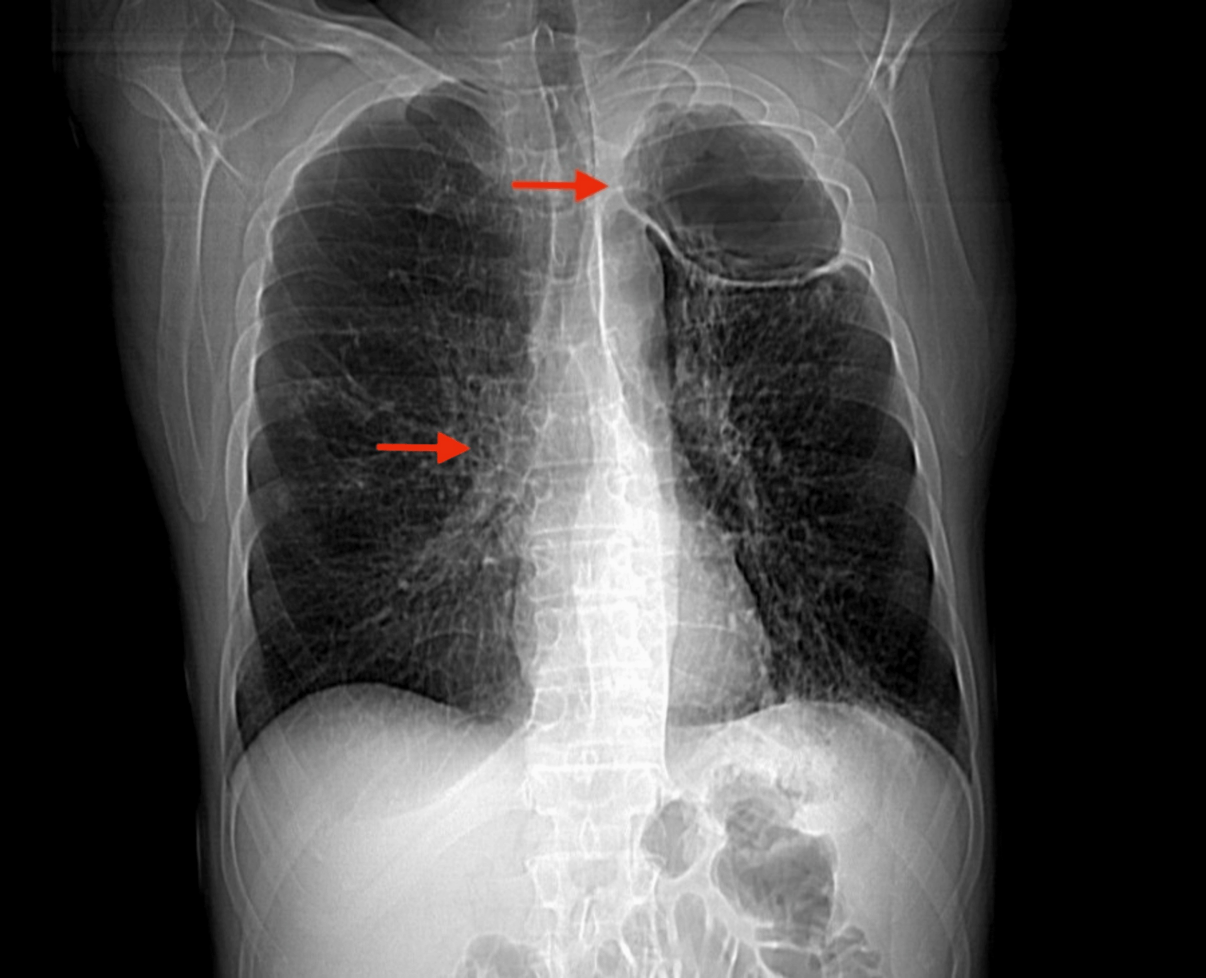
Chest AP erect plain film Chest X-ray with an arrow highlighting a large left apical cavity with nodular wall thickening. A second arrow points to several perihilar and infrahilar nodules.
